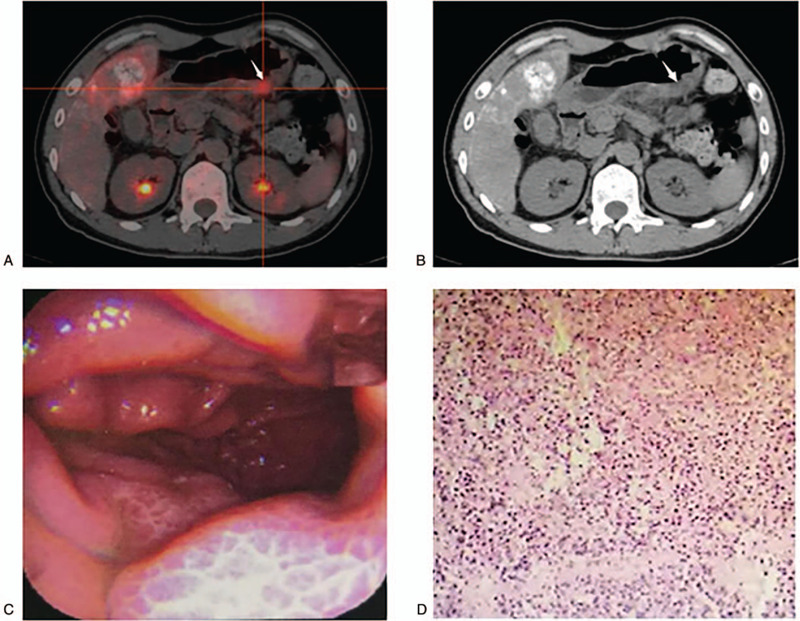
(A) Positron emission tomography/computed tomography fusion image showed a focal abnormal uptake of 2-deoxy-2-(^18^F)-fluorodeoxyglucose in the greater curvature side which was indicated by the white arrow. (B) Focal gastric wall was a bit thicker, and its density was slightly lower on the plain computed tomography image indicated by the white arrow. (C) Gastroscope only showed the local rough slight uplift, it was not easy to distinguish lesion gastric mucosal tissue with naked eyes. (D) Final pathological results suggested neuroendocrine tumor, G3.
